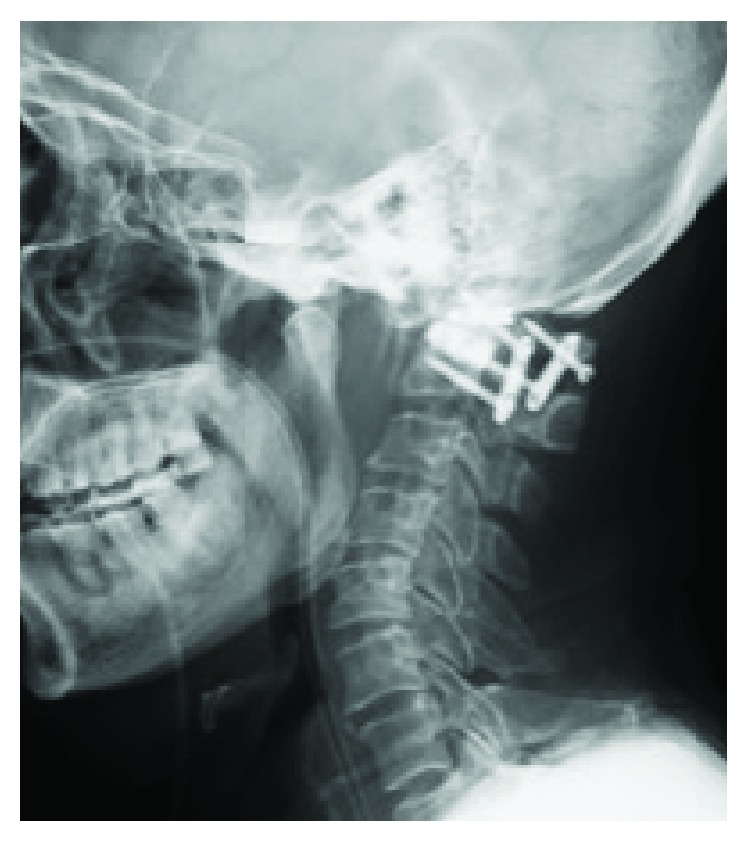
Lateral cervical X-ray at the first visit to our hospital showing instruments on C1/C2, O-C2 angle of 17-degree kyphosis, and vertical subluxation: Redlund-Johnell's distance, 22 mm. Space available for the cord (SAC), atlantodental interval (ADI), and C2-7 angle are 15 mm, 2 mm, and 33°, respectively.
